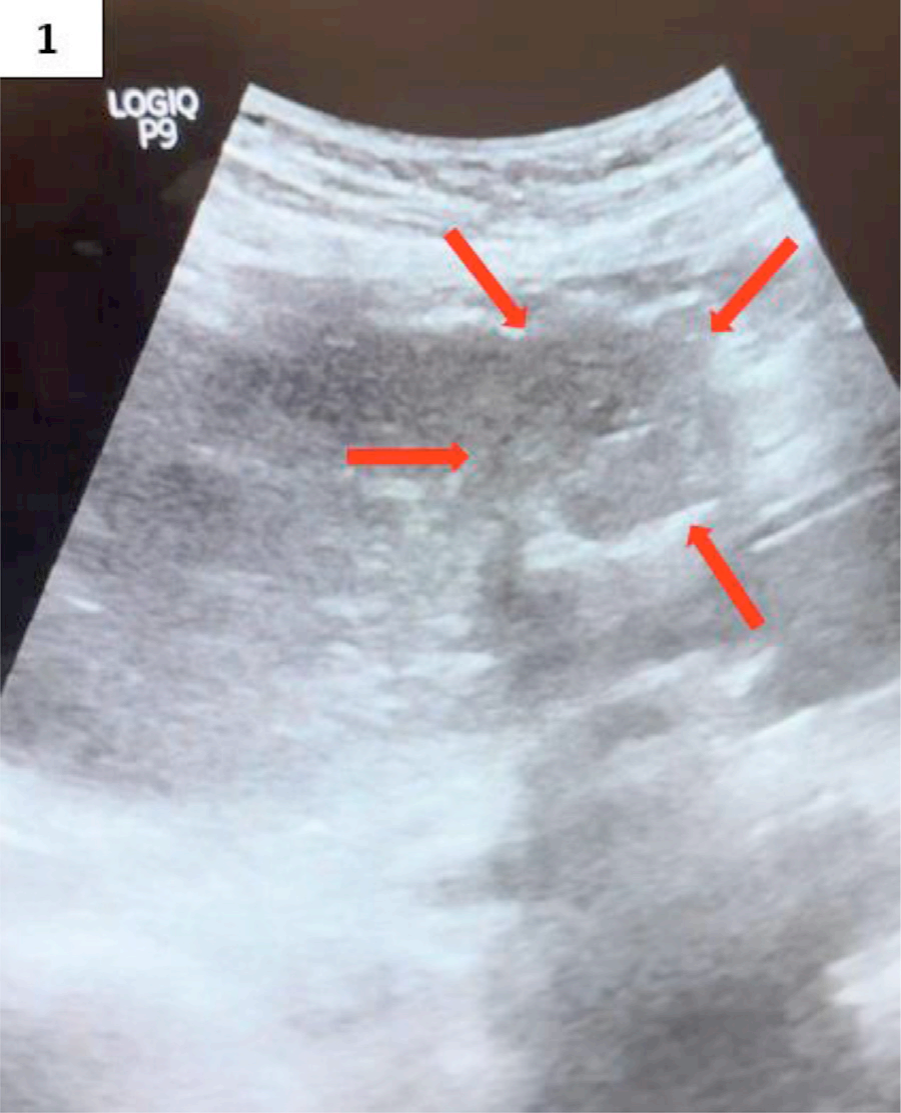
Abdominal ultrasound. Well-defined, oval-shaped mass with regular contours and heterogeneous hypoechoic echotexture, containing calcifications causing posterior acoustic shadowing (red arrows).
